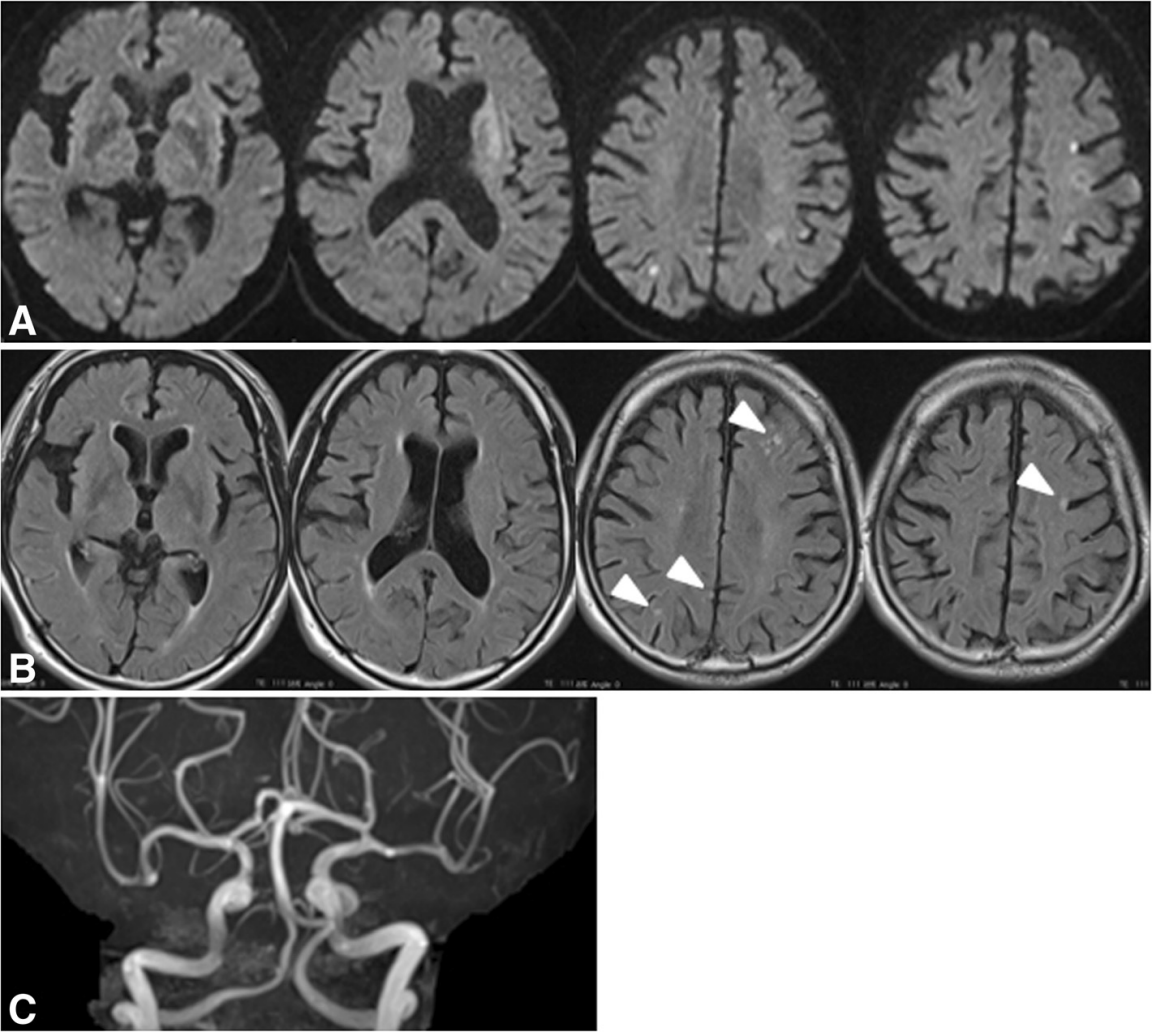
Head magnetic resonance imaging after onset. A: Diffusion-weighted image (DWI) showing a high-intensity area extending from the basal ganglia to the corona radiata of the left cerebrum and multiple small high-intensity areas at bilateral cerebral cortices. B: Fluid-attenuated inversion recovery showing several small high-intensity areas in the same locations detected on DWI (arrowheads). C: Magnetic resonance angiography demonstrating no steno-occlusive changes.
